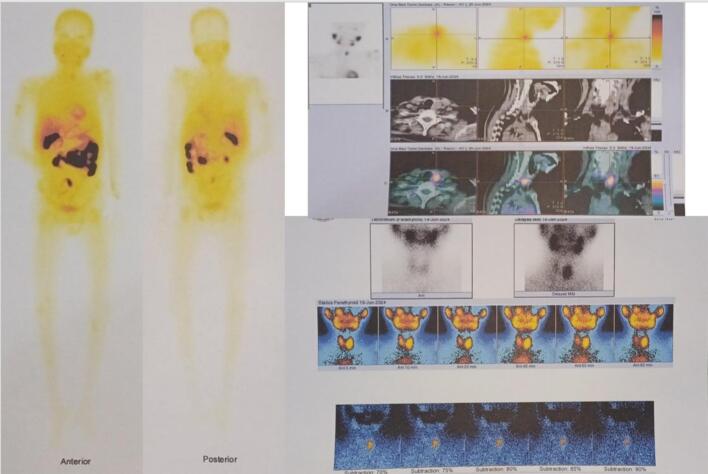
The examination using a whole body scan with ^99m^Tc-*MIBI* showed pathological uptake of radiopharmaceuticals in the projections in multiple sites and *parathyroid scintigraphy* with ^99m^Tc-*MIBI* showed a large parathyroid adenoma located at the level of the left thyroid lobe.
